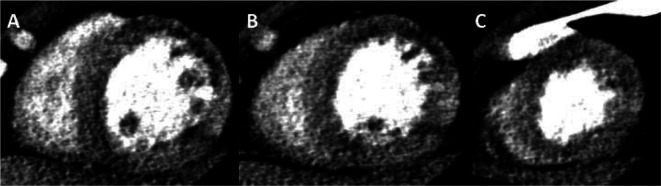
Dipyridamole stress CTA. A-C) Basal, mid and apical short axis reconstructions of a stress CT data set demonstrating an LAD territory perfusion defect in a Kawasaki patient with LAD aneurysm and prior occlusion.
